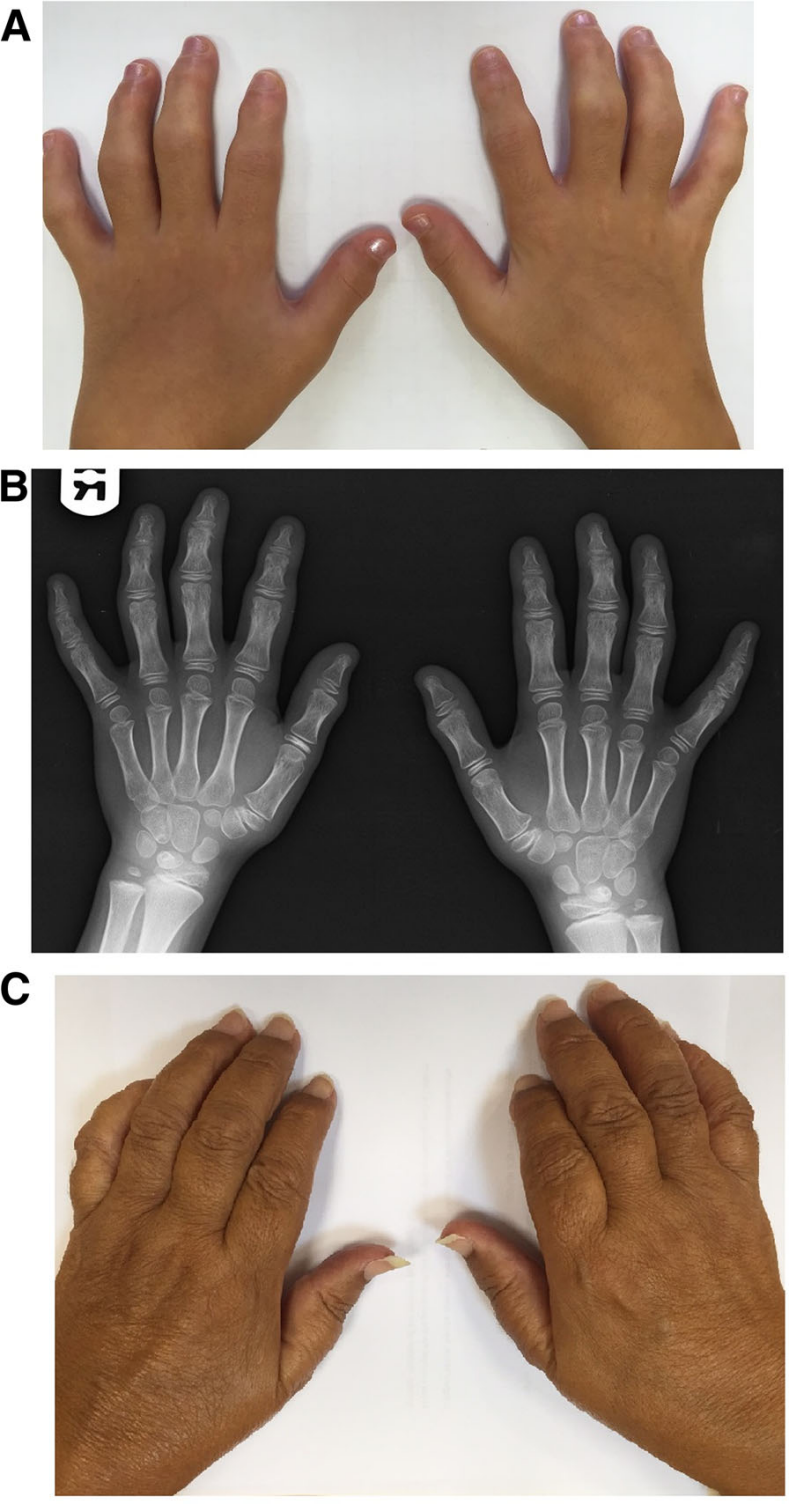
Clinical and radiographic findings in Progressive Pseudorheumatoid Dysplasia. a Bilateral symmetric bony enlargements of the 1st interphalangeal joints of patient V:1 (at the age 9.5 years). b Radiograph of patient V:1 (at age 6.5 years) demonstrating interphalangeal joint metaphyseal enlargement. c Camptodactyly with reduced range of motion of multiple hand joints, and bilateral bony enlargement of the interphalangeal joints of patient III:13 (at the age of 53 years)
